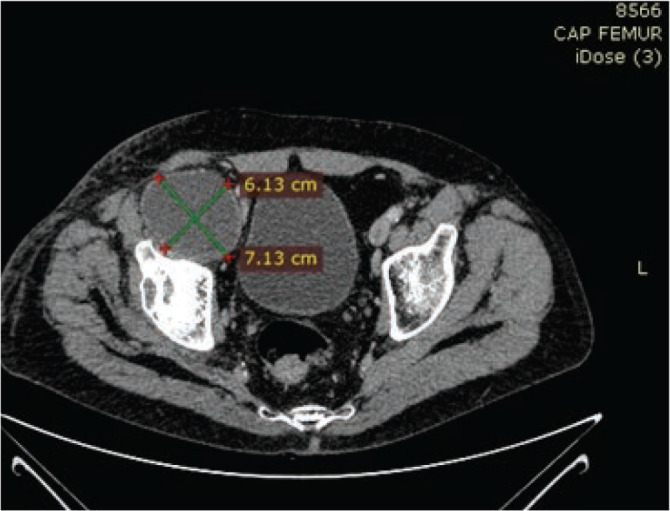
CT scan with IV contrast showing cystic lesion with contrast enhancement of its thin wall in conjunction with the right hip joint in front of the iliopsoas muscle.
